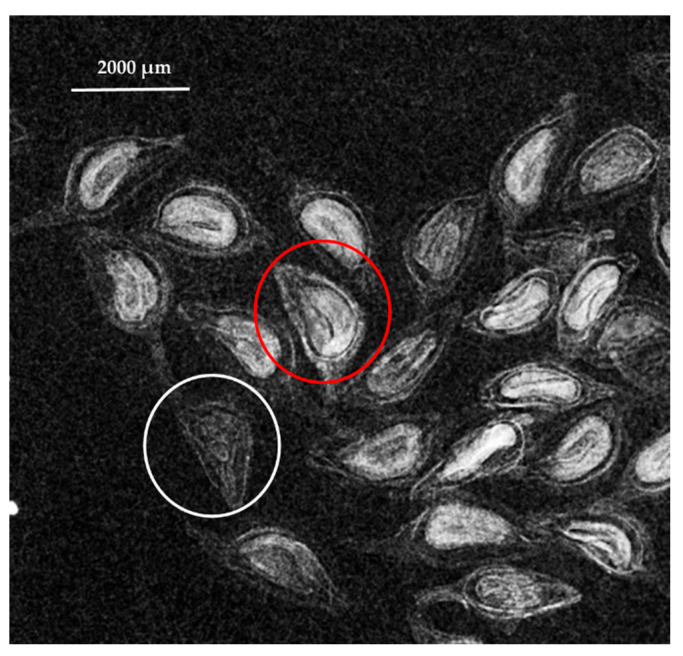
Seeds of *S. platyphylla* under X-ray examination (Faxitron MX-20), with the seed circled in red identified as filled and the seed circled in white considered to be unfilled.
